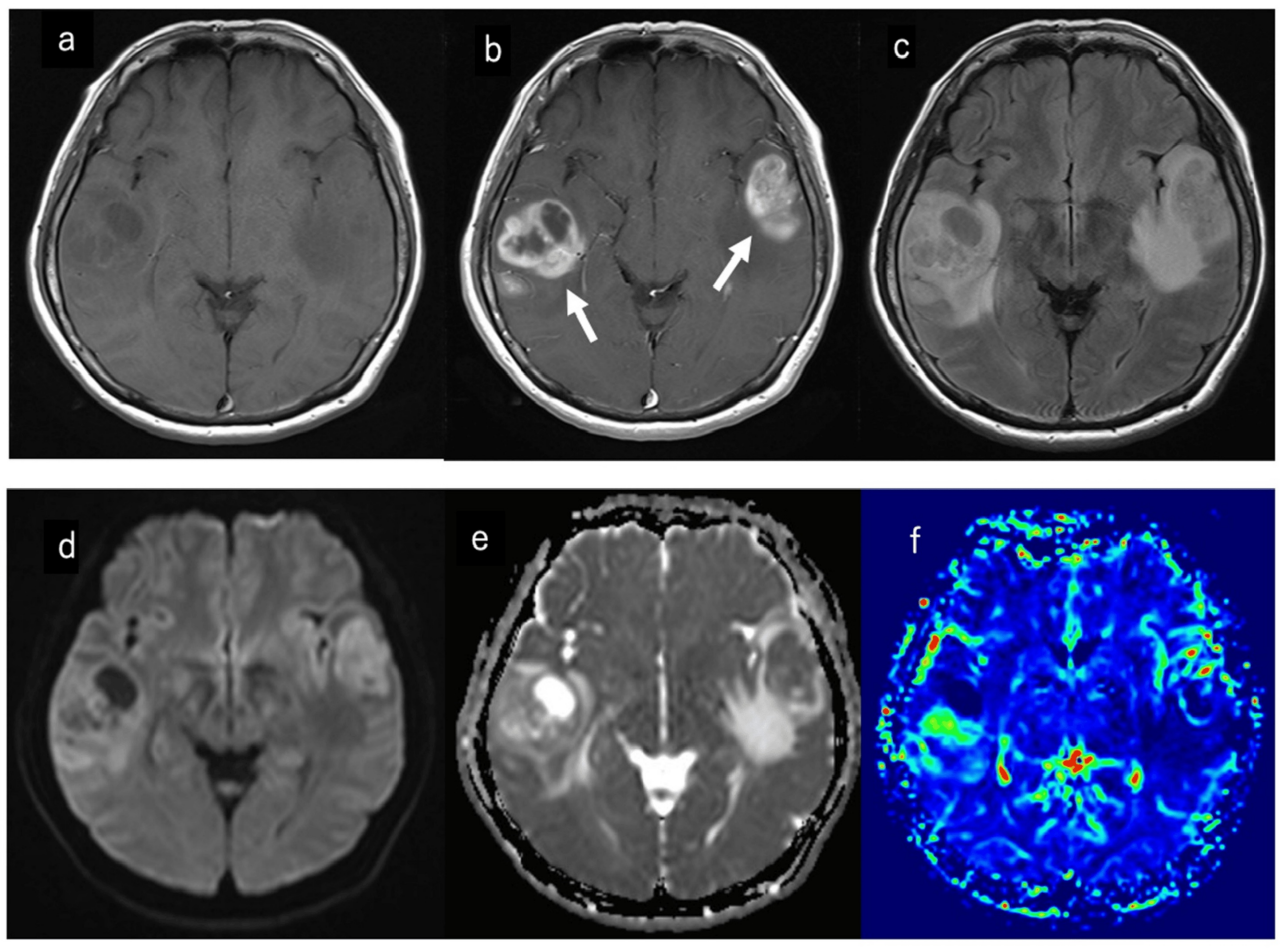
Preoperative brain MRI scans. (a) Preoperative T1-weighted MRI (axial). (b) Preoperative gadolinium-enhanced T1-weighted MRI, with white arrows indicating the tumors. (c) Preoperative FLAIR image (axial). (d) Preoperative DWI (axial). (e) Preoperative ADC image (axial). (f) Preoperative rCBV image (axial). FLAIR: fluid-attenuated inversion recovery; rCBV: relative cerebral blood volume; DWI: diffusion-weighted imaging; MRI: magnetic resonance imaging.
